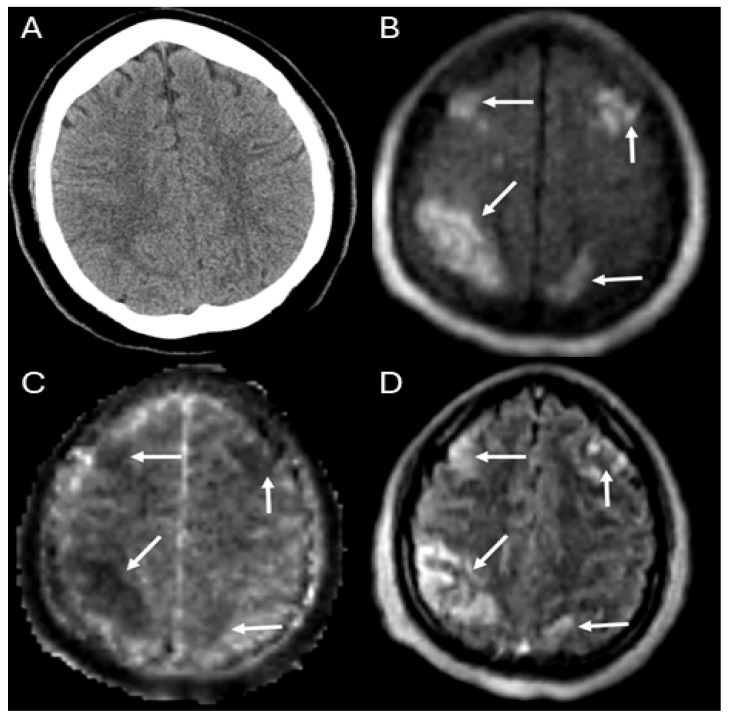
Example case: 31-year-old recently postpartum female with thrombotic thrombocytopenic purpura requiring extracorporeal cardiopulmonary resuscitation after cardiac arrest whose ULF-pMR demonstrated multifocal ischemic stroke (arrows: bilateral frontal and parietal strokes) on the Panels (B) (DWI), (C) (ADC), and (D) (FLAIR), not seen on the HCT (Panel (A)).
